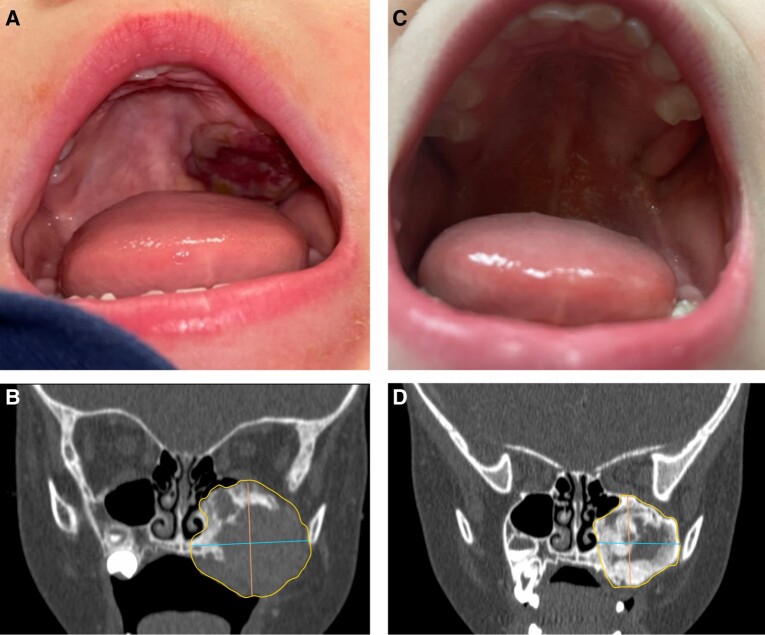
Representative images of denosumab treatment in central giant cell granuloma. The left-hand panels show baseline images from a 2-year-old boy. This includes a photograph showing an expansile palatal mass (A) and computed tomography demonstrating an expansile lytic lesion of the maxilla with thinned cortices and areas of cortical destruction (B). The right-hand panels show response after denosumab treatment, including regression of the palatal mass (C) and progressive peripheral sclerosis with increased radiodensity (D).
